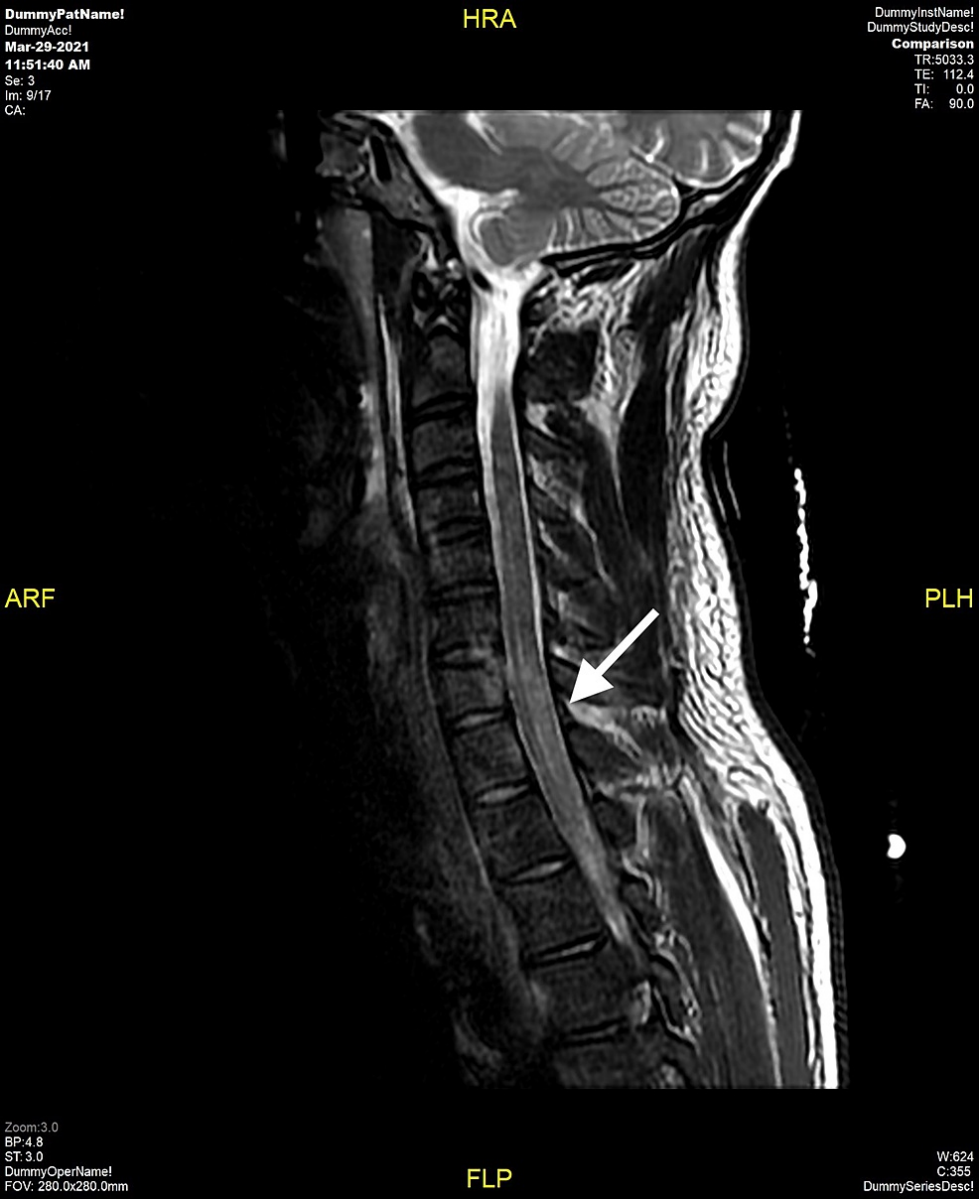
MRI of the Cervical Spine Showing Indistinct Edema from C5 to T1 (arrow)
